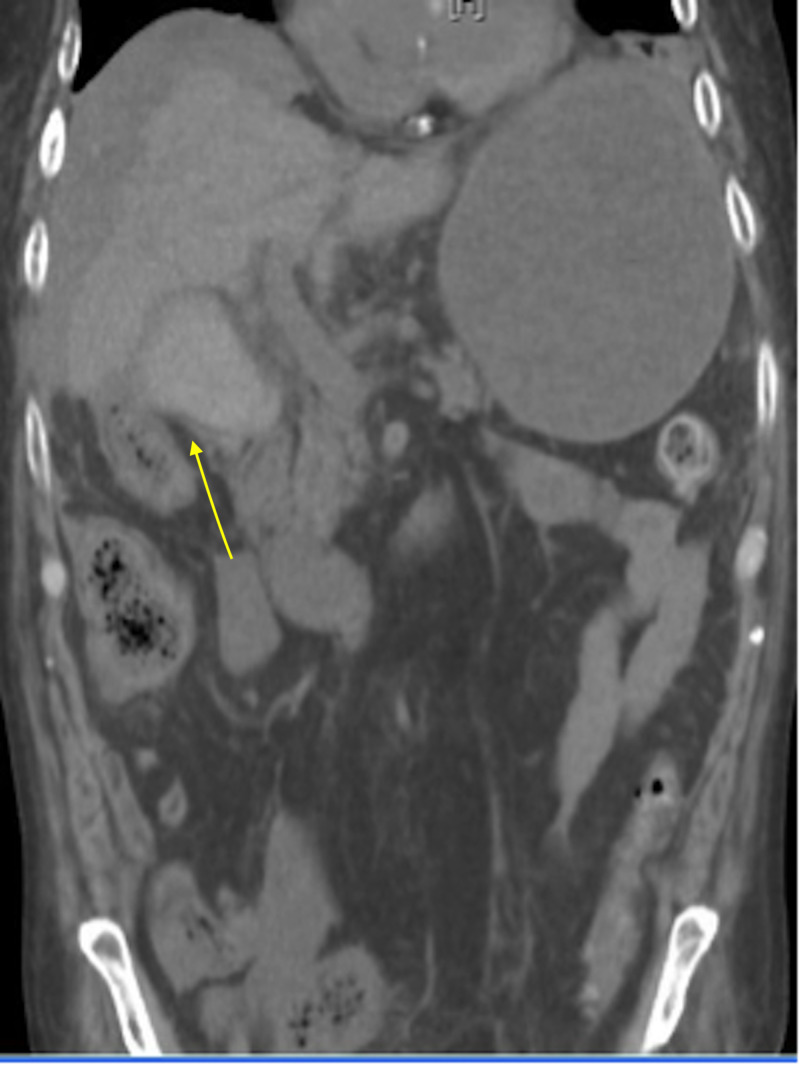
CT of the abdomen and pelvis without contrast Hemorrhage within the gallbladder resulting in a subcapsular hematoma
